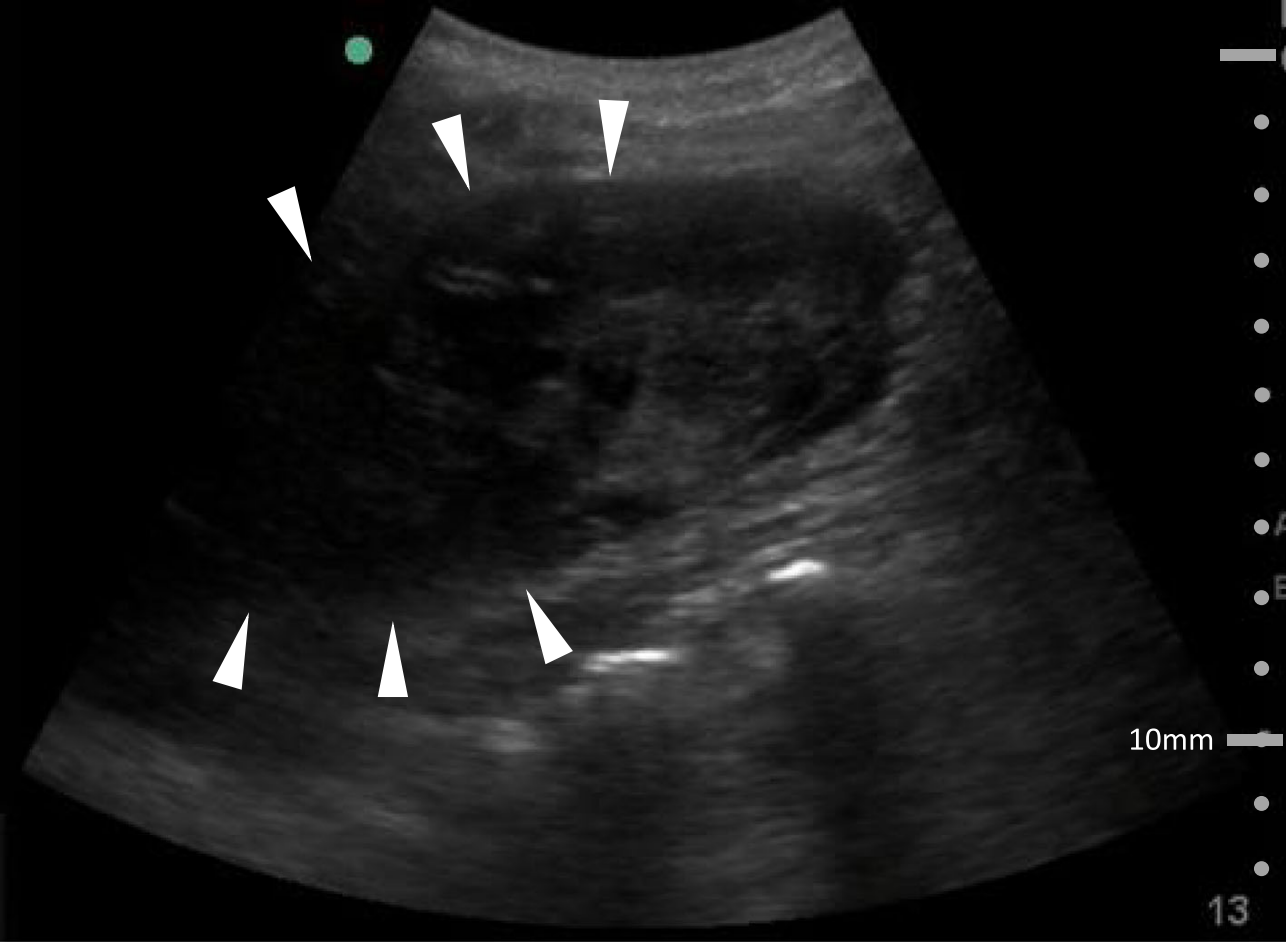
Ultrasound image of the left first lumbar level. Hypoechoic area (arrow head) suggestive of hematoma was detected below the trigger point injection site observed in the second emergency room. The renal parenchyma is a slightly hyperechoic area to the right of the renal hematoma
